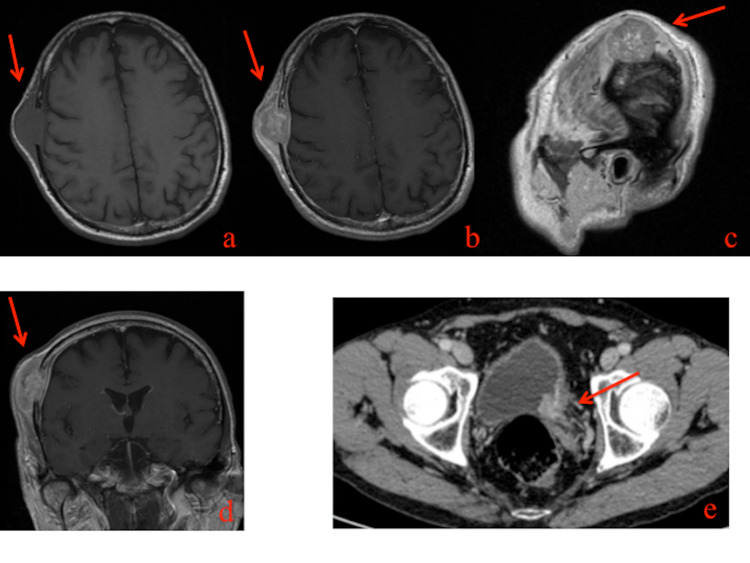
Preoperative MRI and abdomen CT scan Pre-contrast T1 axial (a), post-contrast T1 axial (b), post-contrast T1 sagittal (c), and post-contrast T1 coronal (d) MRI sequences documented an expansive lesion (28x20x38mm diameter) of the right frontotemporal cranial theca. The lesion caused complete lysis to the thickness of the cranial bone. Post-contrast abdomen CT scan showed the presence of a left postero-lateral bladder wall lesion (e).
